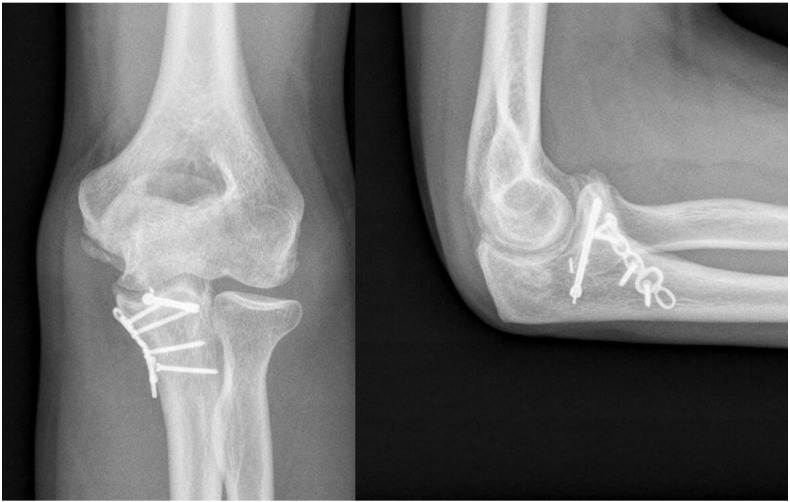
Postoperative radiographic exam at 2 years of follow-up shows good healing of the fracture and good alignment of the elbow. The patient has good motion and has returned to his pre-injury level of activity with no restrictions.
